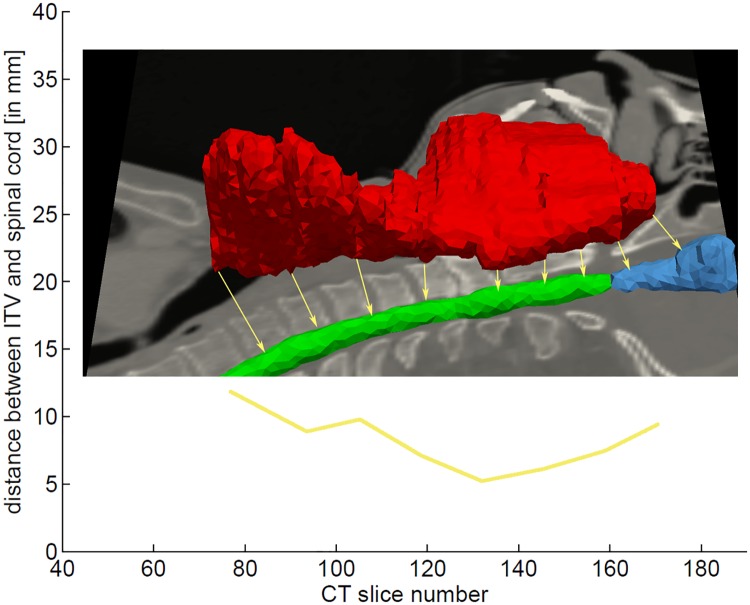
Distance between ITV and OARs per CT slice. The slice wise distance between ITV and spinal cord *d*_SC_(*z*) is used to estimate the risk to the spinal cord.
